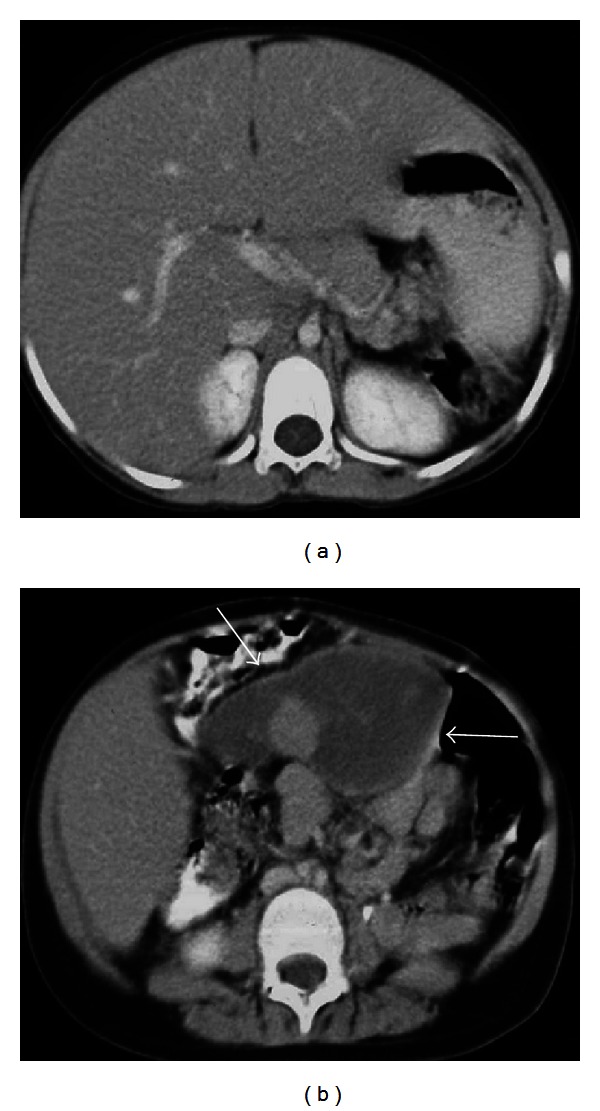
Axial contrast enhanced CT images of a 19-year-old female patient who was presented with acute abdominal pain. There is no splenic tissue at left upper quadrant (a) and a heterogenously hypodense mass (arrows) of a torsioned wandering spleen at midline (b) which was proven surgically. Hypodense parenchyma indicates infarction.
